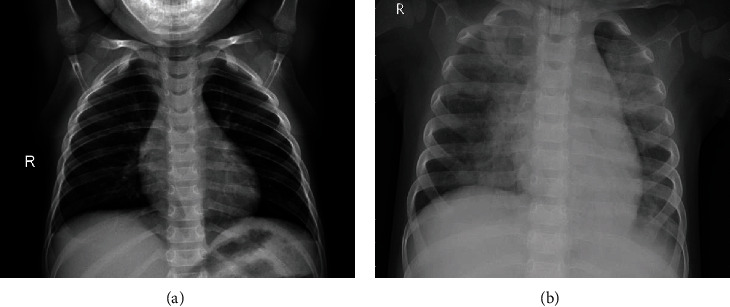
Samples of chest X-ray images from prepared dataset. (a) Normal. (b) COVID-19.
